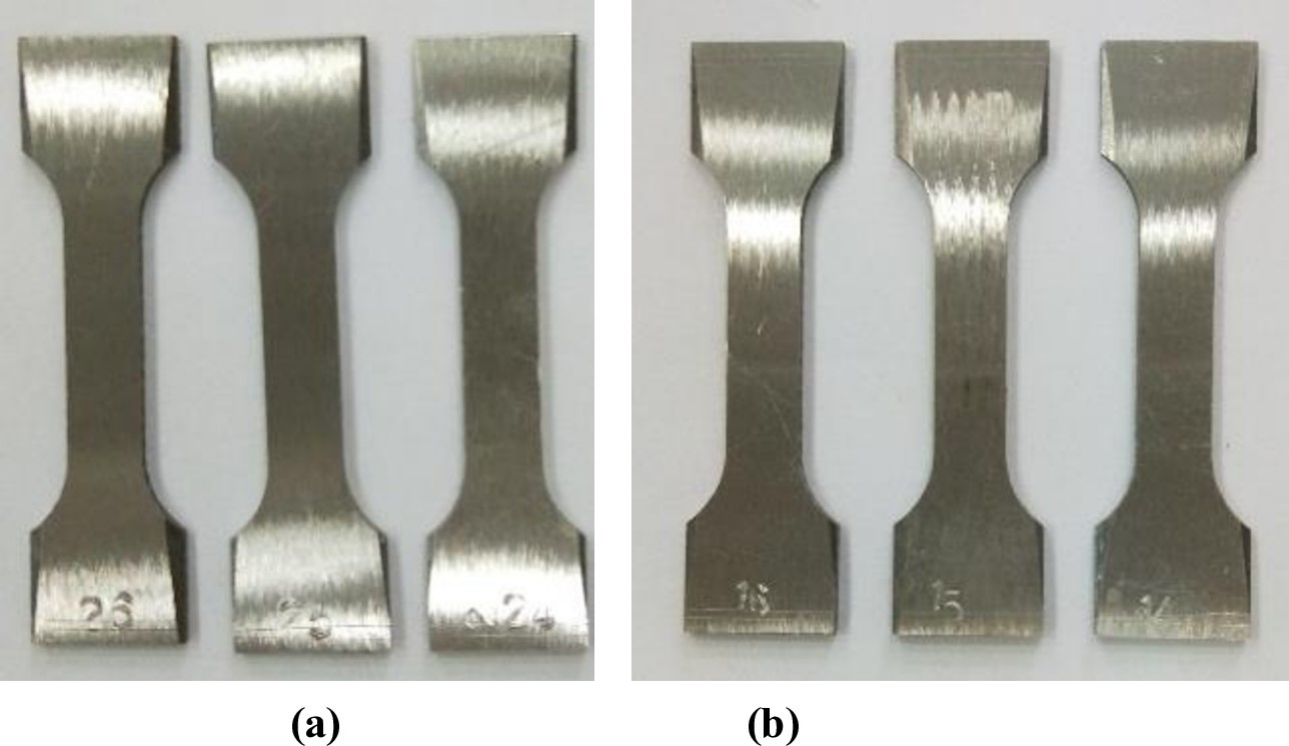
Standard tensile test samples of the WM and BM of CT. (a) WM, (b) BM.
